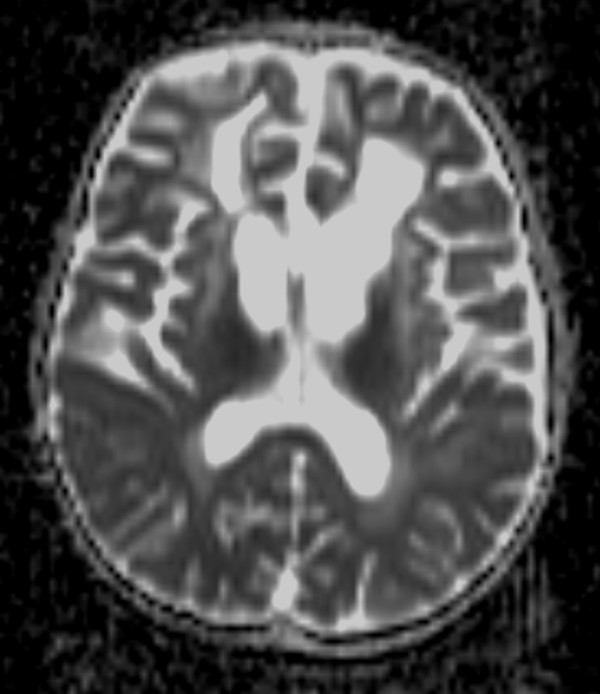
Magnetic resonance imaging at 5 years, 4 months. a T2-weighted image shows increase in abnormal signals in the frontal lobe and volume loss in bilateral white matter and deep gray matter. b T2-weighted image demonstrates an increase in abnormal signals in the medulla oblongata. c Fluid attenuated inversion recovery image shows swelling and high intensity from the medulla oblongata to the top of the cervical spine. d, e Mild elevation in apparent diffusion coefficient values are recognized in affected areas.
